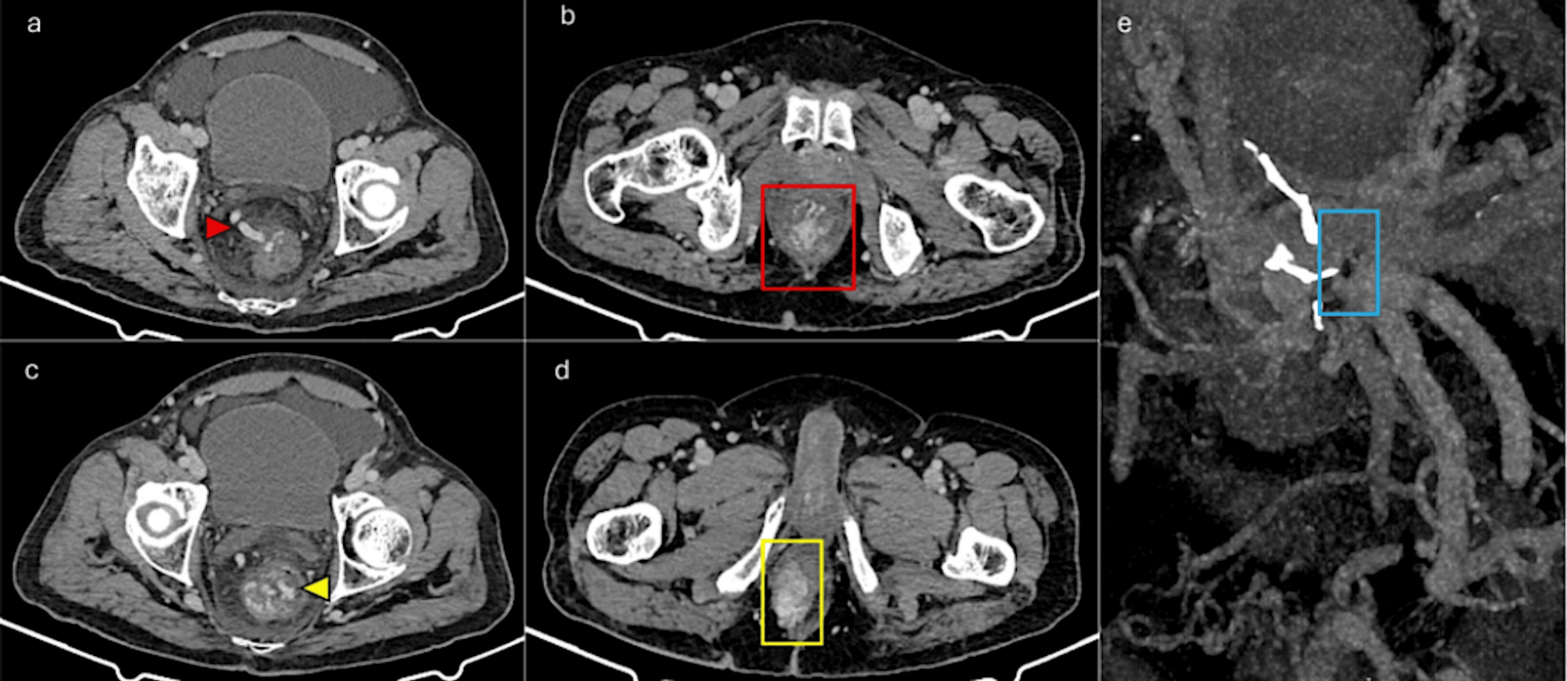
Pre-procedure computed tomography image The image shows (a) a dilated and tortuous IMV (red arrowhead) supplying the rectal varices in (b) (red box); (c) active extravasation (yellow arrowhead) and prolapse of the ano-rectal complex are also apparent (d) (yellow box). (e) CT images show stigmata of portal hypertension with severe ostio-proximal stenosis of the SMV and IMV (blue box). These were later confirmed with diagnostic digital subtraction angiography. IMV: inferior mesenteric vein; SMV: superior mesenteric vein; CT: computed tomography
